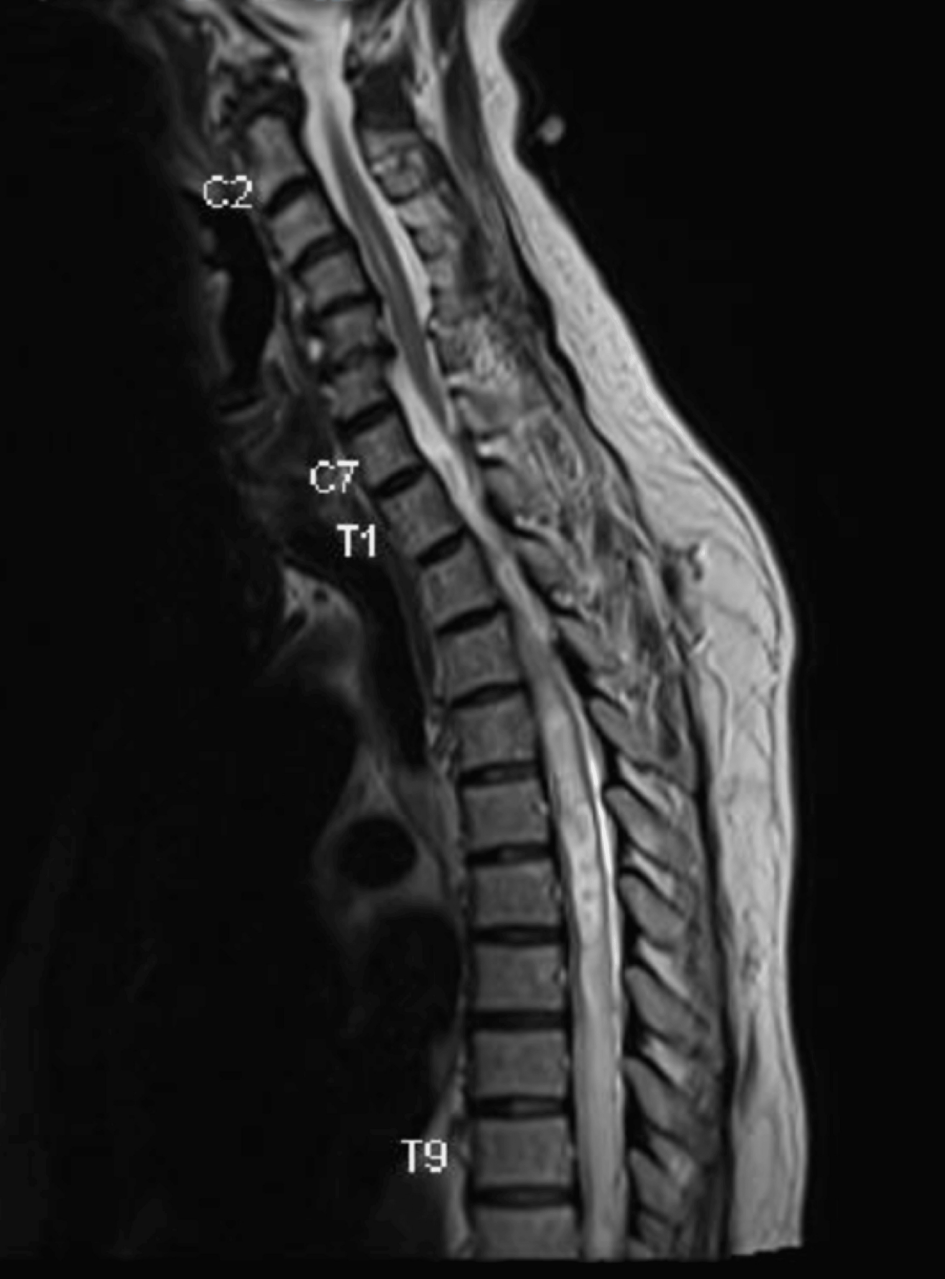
Pre-operative T2-weighted sagittal MRI image showing extensive syrinx.
